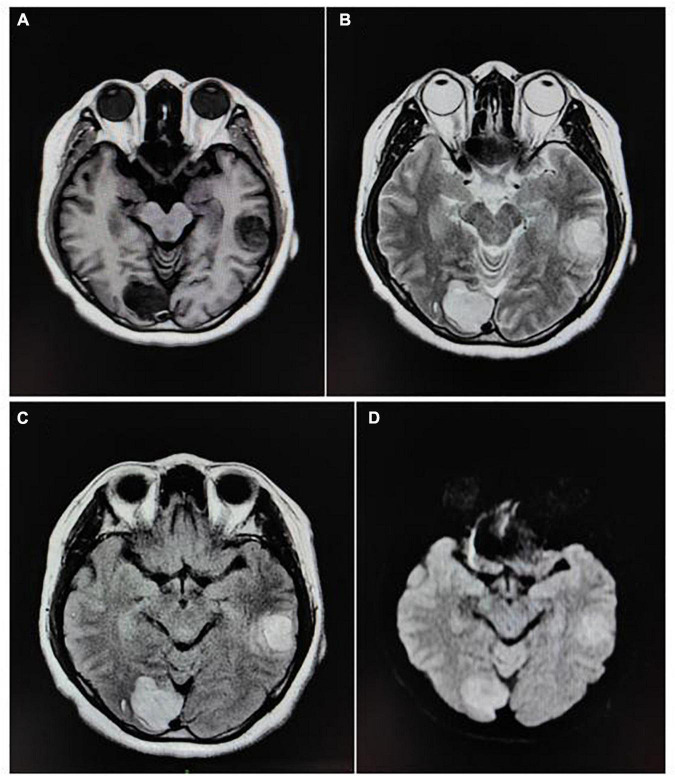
MRI results of MTB. The patient, a 57-year-old woman, presented with MTB in the left temporal lobe and right occipital lobe. The primary tumor was lung cancer, single and irregular. (A) Transverse T1WI. (B) Transverse T2WI. (C) Transverse T2 FLAIR. (D) Transverse DWI.
